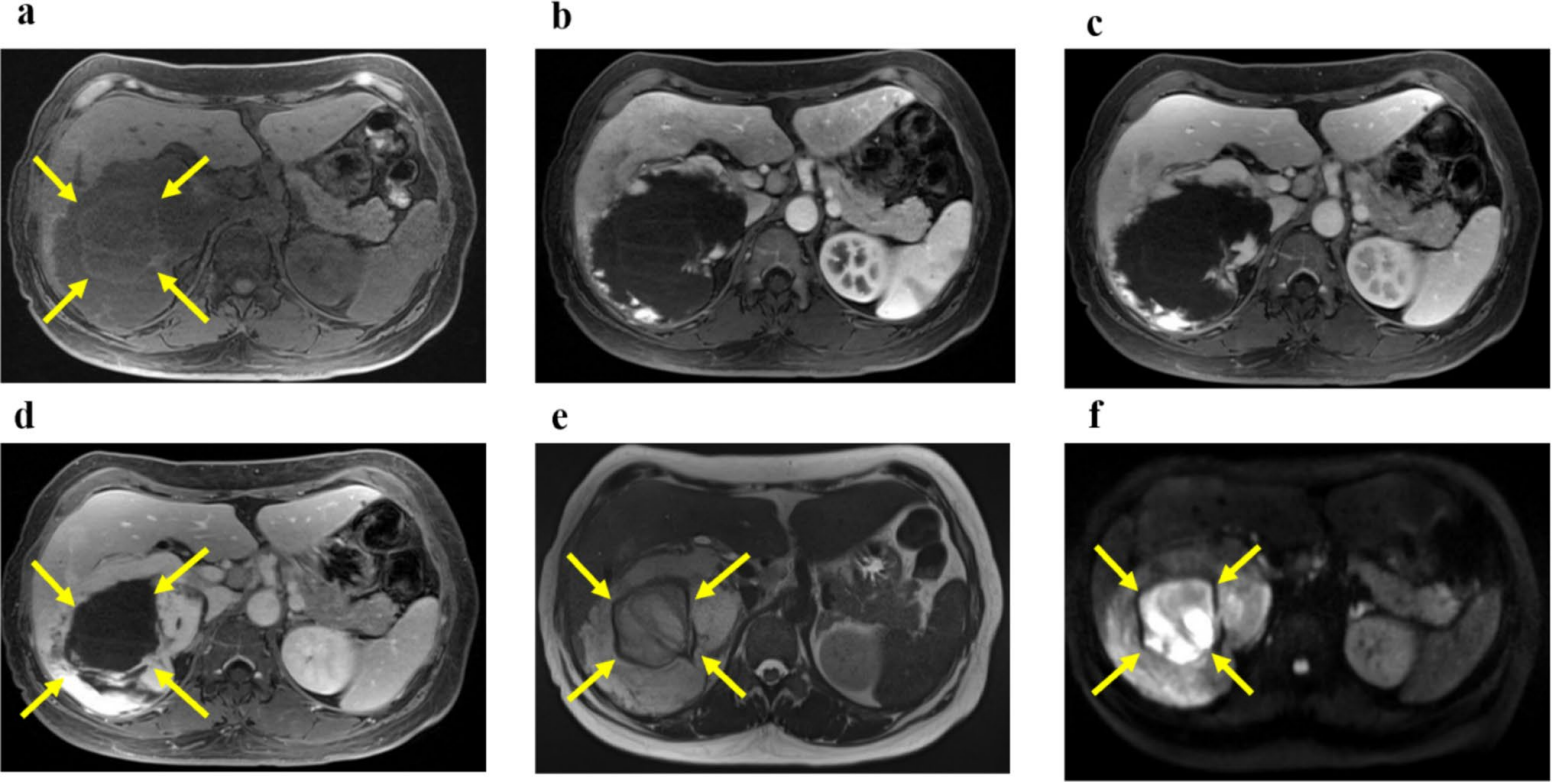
Abdominal MRI. T1 weighted-enhanced images a. Artery phase b. Portal phase c. Delay phase d. T2 weighted-enhanced image e. Diffusion-weighted image b=800 f. A giant hemangioma, 130 mm in size, was observed in the posterior right lobe of the liver and contrasted with the periphery. The interior of the hemangioma showed a well-defined non-contrast area, which appeared as an abnormal signal area in the diffusion-weighted image. MRI, magnetic resonance imaging. The yellow arrows indicate areas of hemorrhage and necrosis within the giant hemangioma
